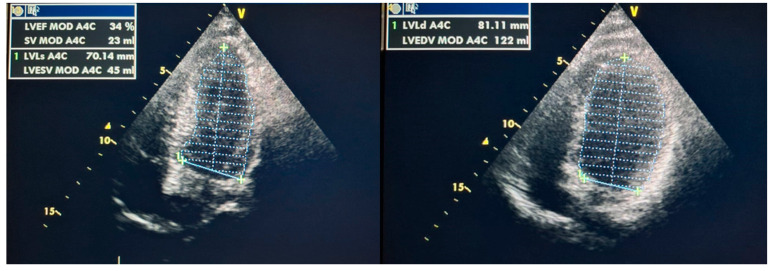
Severe dysfunction of left ventricular function in echocardiography with EF = 34% calculated using the Simpson method.
